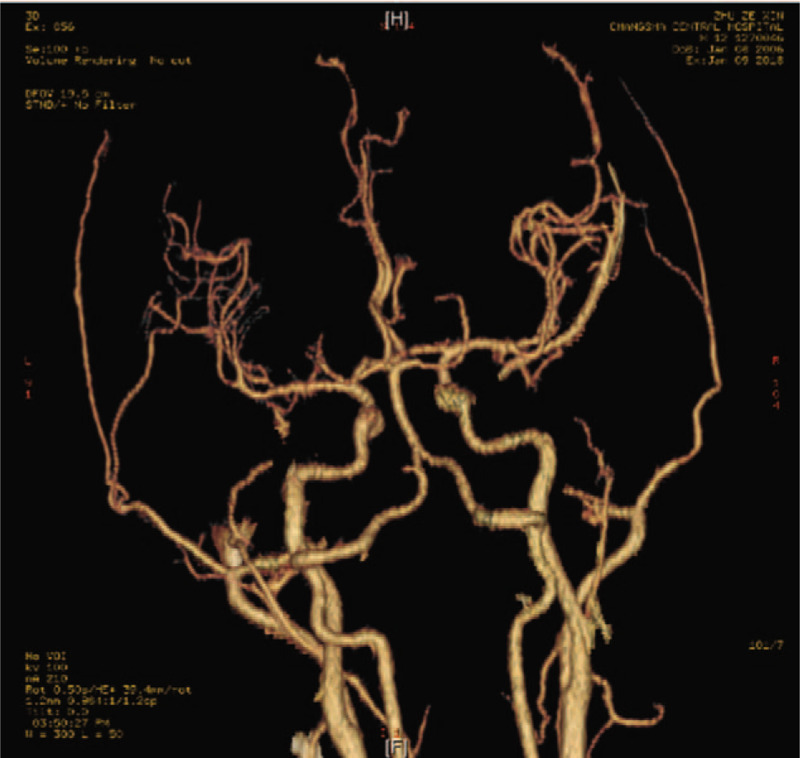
Brian computed tomography angiography (CTA; January 9, 2018). Blood flow of right internal carotid artery was restored, and the right middle cerebral artery and the anterior cerebral artery were demonstrated normally.
